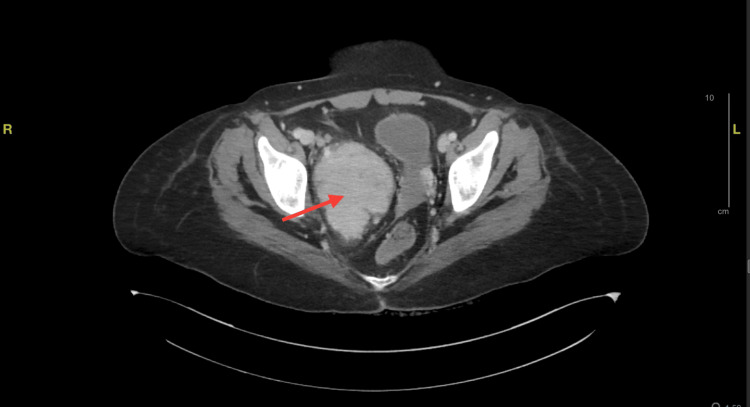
CT scan showing single large abdominal pelvic mass (red arrow), and multiple retroperitoneal and pelvic lymphadenopathy.
